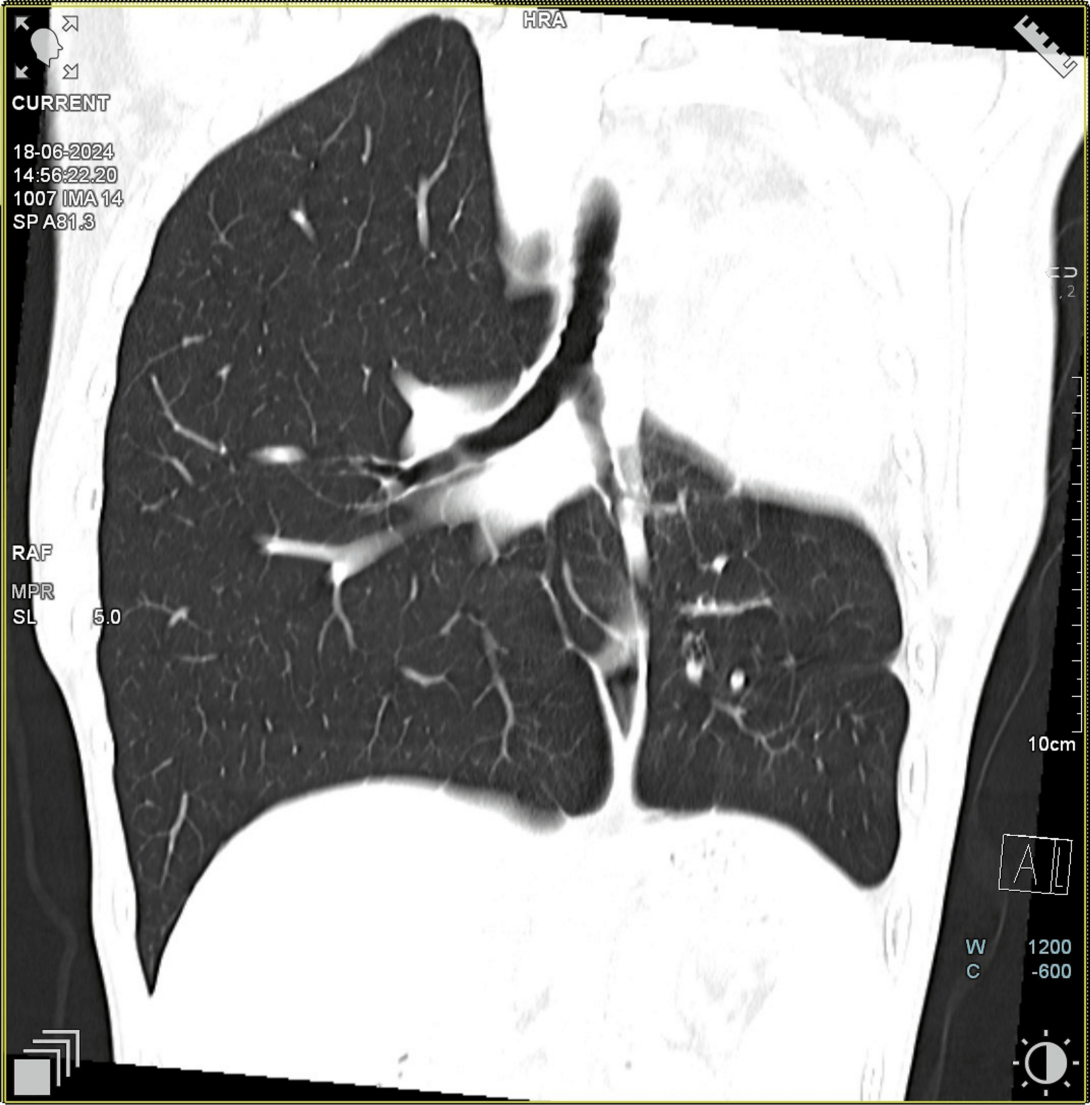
CT thorax coronal section The image shows the absence of the left upper lobe bronchus and the left upper lobe. The left main bronchus continues as the left lower lobe bronchus CT: computed tomography
